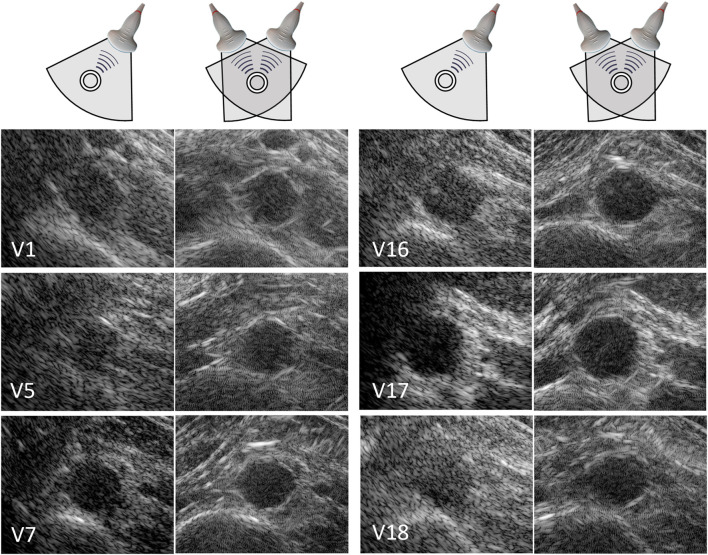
Single-perspective (left), and coherent dual-aperture bistatic (right) images of the abdominal aorta in 6 volunteers (V1, V5, V7, V16, V17, and V18). All ultrasound images are displayed using a 50 dB dynamic range. The size of the zoomed windows are 5 cm in the x-direction and 4 cm in the z-direction.
